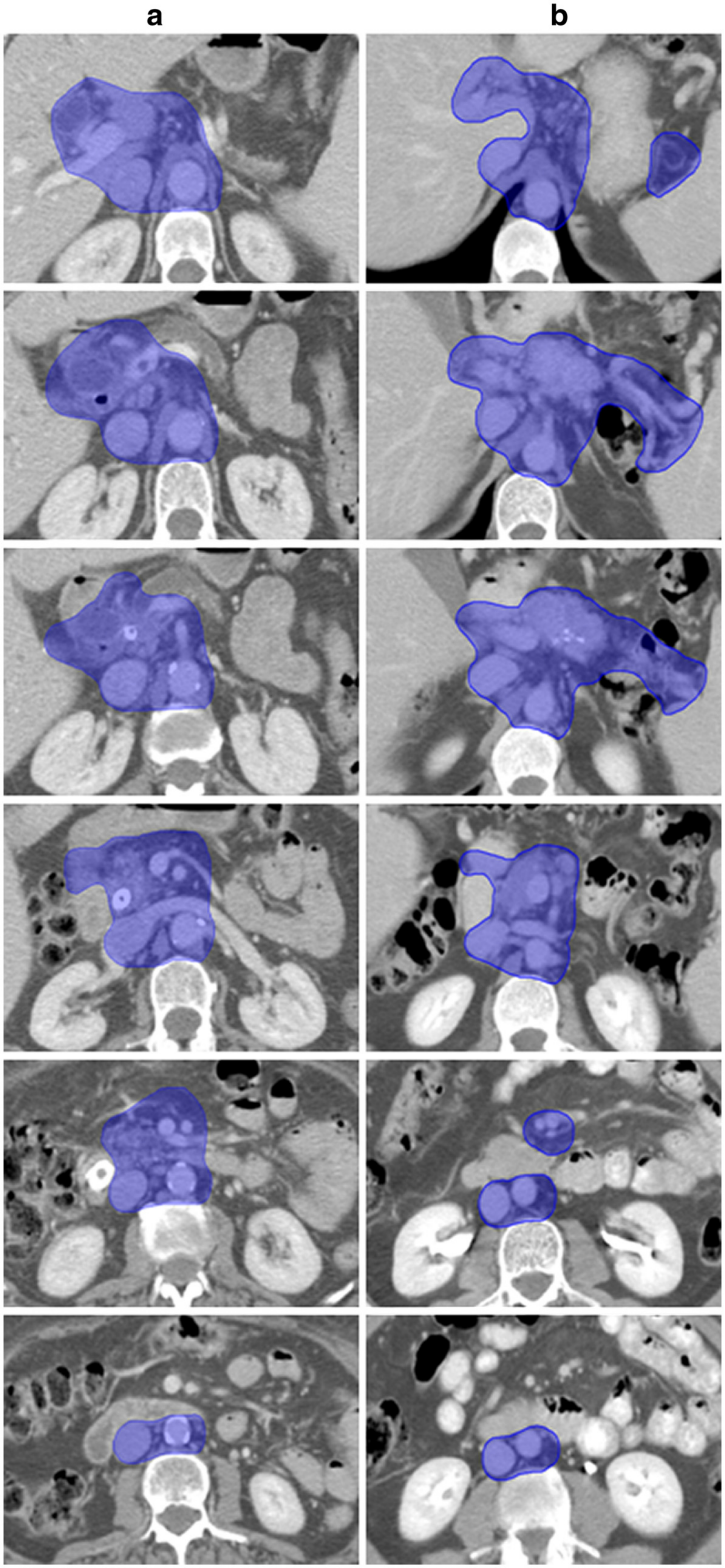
CTV delineation in the treatment of the head and the body pancreas adenocarcinoma. Transverse CT slices through high risk lymph node regions of the head (a) and body/tail (b) pancreatic cancer from cranial to caudal direction. CTV was formed by the union of the identified lymph node areas (blue area).
